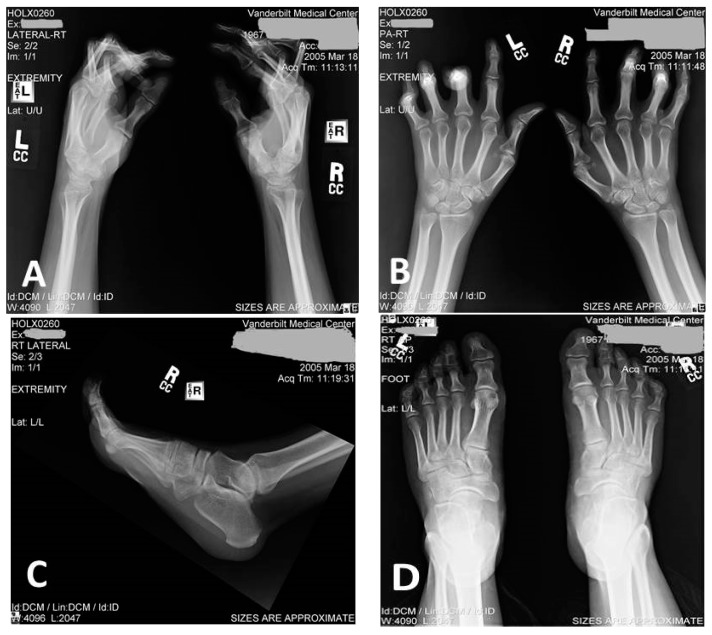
Radiograph of proband’s hands (A = lateral view; B = anterio-posterior (A/P view) and feet (C = lateral R foot and D = A/P both feet).
